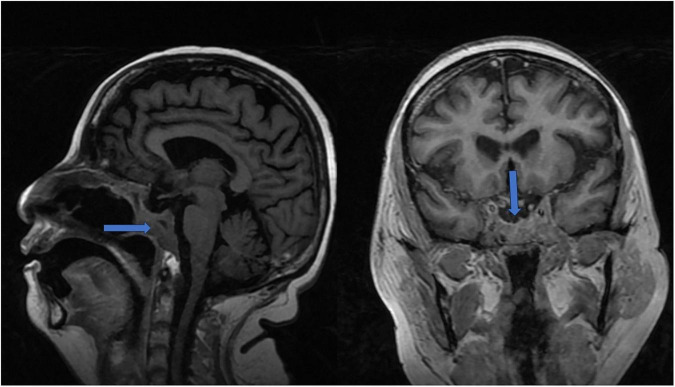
Sagittal T1-weighted MRI showing pituitary macroadenoma/Coronal T1-weighted MRI showing pituitary macroadenoma with sphenoid sinus extension.
